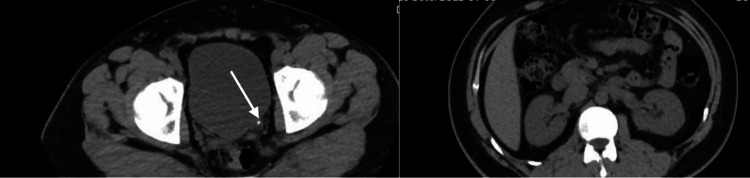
Noncontrast computed tomography of the urinary tract demonstrating a 4 mm calculus at the left ureterovesical junction (arrow), without hydronephrosis
